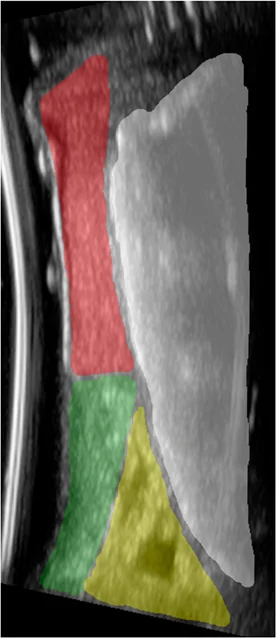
Cross‐sectional anatomical scan of the alveolar ridge. The sound wave reflects strongly at the bone surface, beyond which there are little or no reflected signals. Therefore, the bone is depicted in white color. In the soft tissue, this ultrasound b‐mode image highlights three different anatomical regions of interest: (1) The keratinized mucosa (KM), characterized by a bright (hyperechoic) surface (salmon color), (2) the lining mucosa, which is apical to the KM with a less bright surface (green color), and (3) the muscle fibers with hypoechoic, striated features (yellow color).
